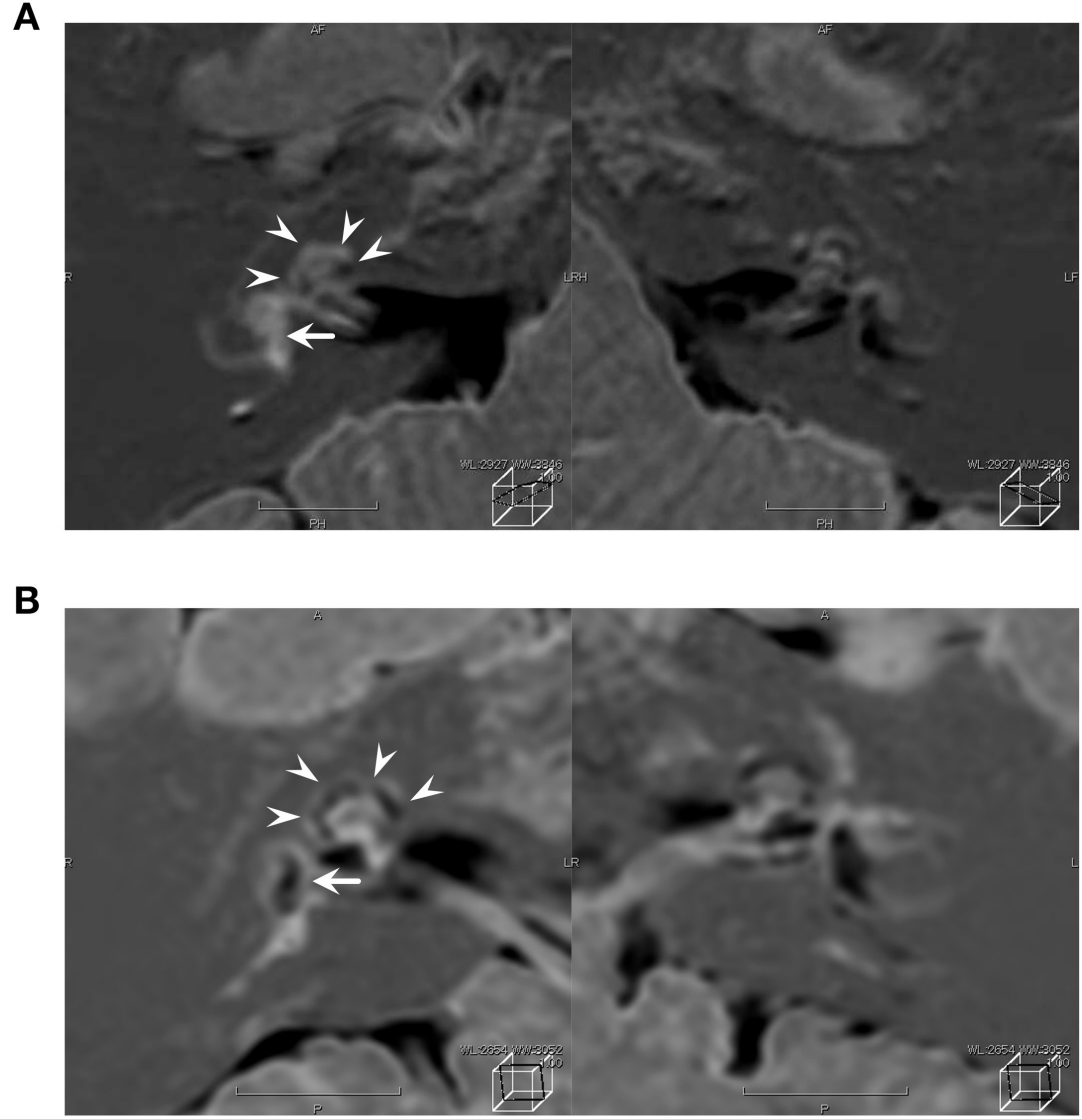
Contrast-enhanced MRI images using HYDROPS. The arrow heads and long arrows indicate the enlarged cochlea vestibule, respectively. Contrast-deficient images (black areas) indicate the presence of endolymph. A contrast-enhanced deficient image of the endolymph of the right ear was rarely observed preoperatively (A) but is observed postoperatively (B).
